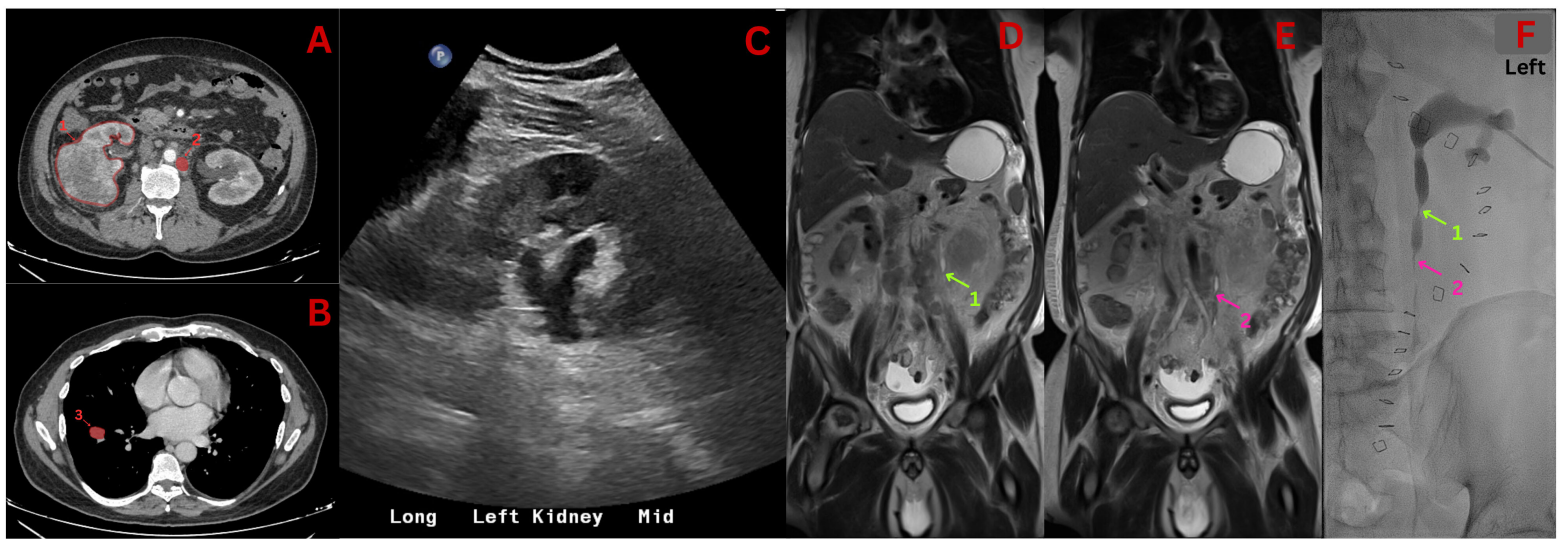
Radiographic imaging results. (A) CT scan revealing right sided renal mass (1) and an enlarged lymph node associated with periureteral stranding (2). (B) CT scan revealing lung metastasis (3). (C) Renal Ultrasound Scan revealing mild hydronephrosis. (D, E) Coronal MRI scans done near nephrostomy tube insertion, showing proximal ureteral obstruction (1 - green) and more distal obstruction (2 - pink). (F) Fluoroscopy revealing ureteric obstruction during nephrostomy tube insertion, correlated with arrows and numbers in figures (D, E).
